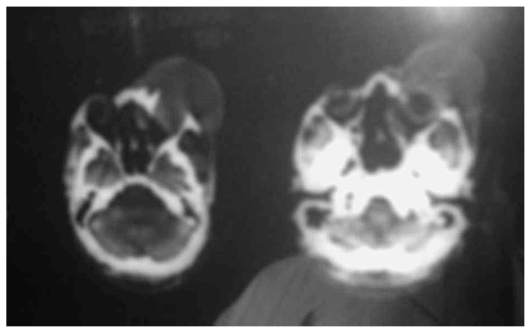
Cranial CT scan of the patient with right sided forehead and ocular adnexal mass showing normal ocular dimensions without any intracranial connection.
